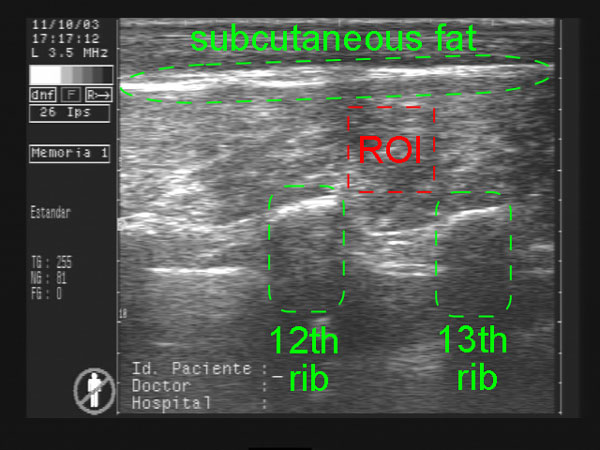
IMF% estimation on ultrasound images. Sample US image.
